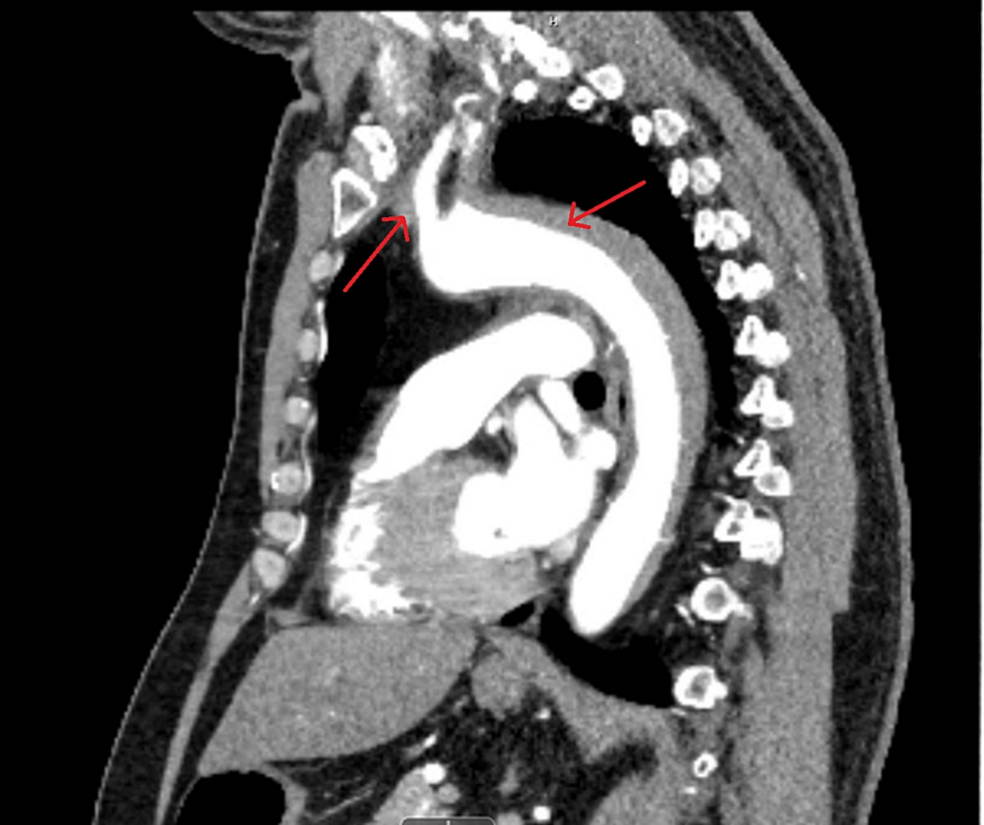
CT imaging showing a sagittal view of the intramural hematoma tracking proximally from the arch of the aorta and distally (red arrows).
